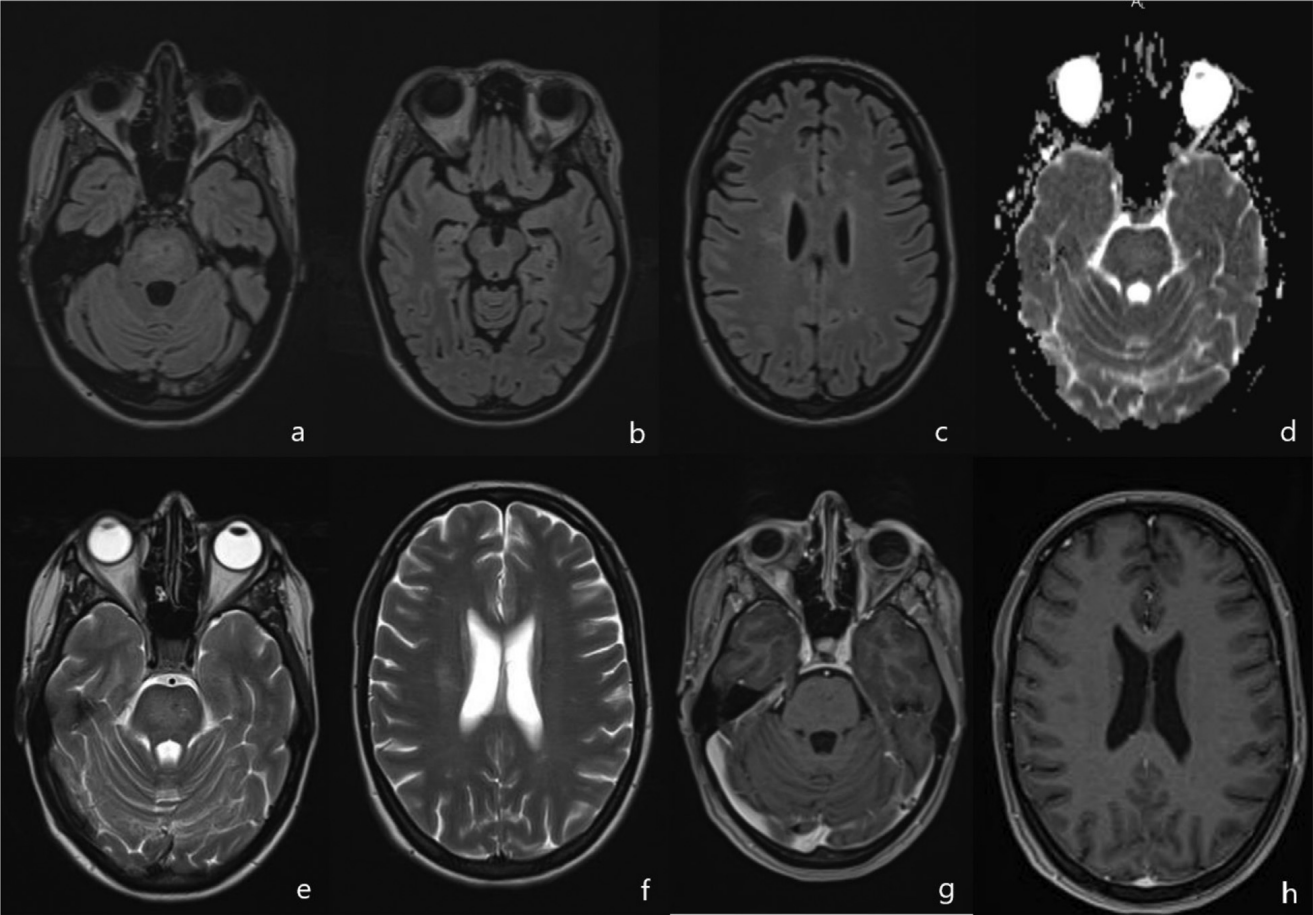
Representative MRI data after treatment with steroids (27 December 2021): FLAIR (a–c), ADC-map (d), T2 (e, f), and the corresponding T1 post gadolinium image (g, h) revealing almost complete resolution of the FLAIR signal aberration, of pontine enlargement, and of contrast enhancement after the brief course of steroids.
